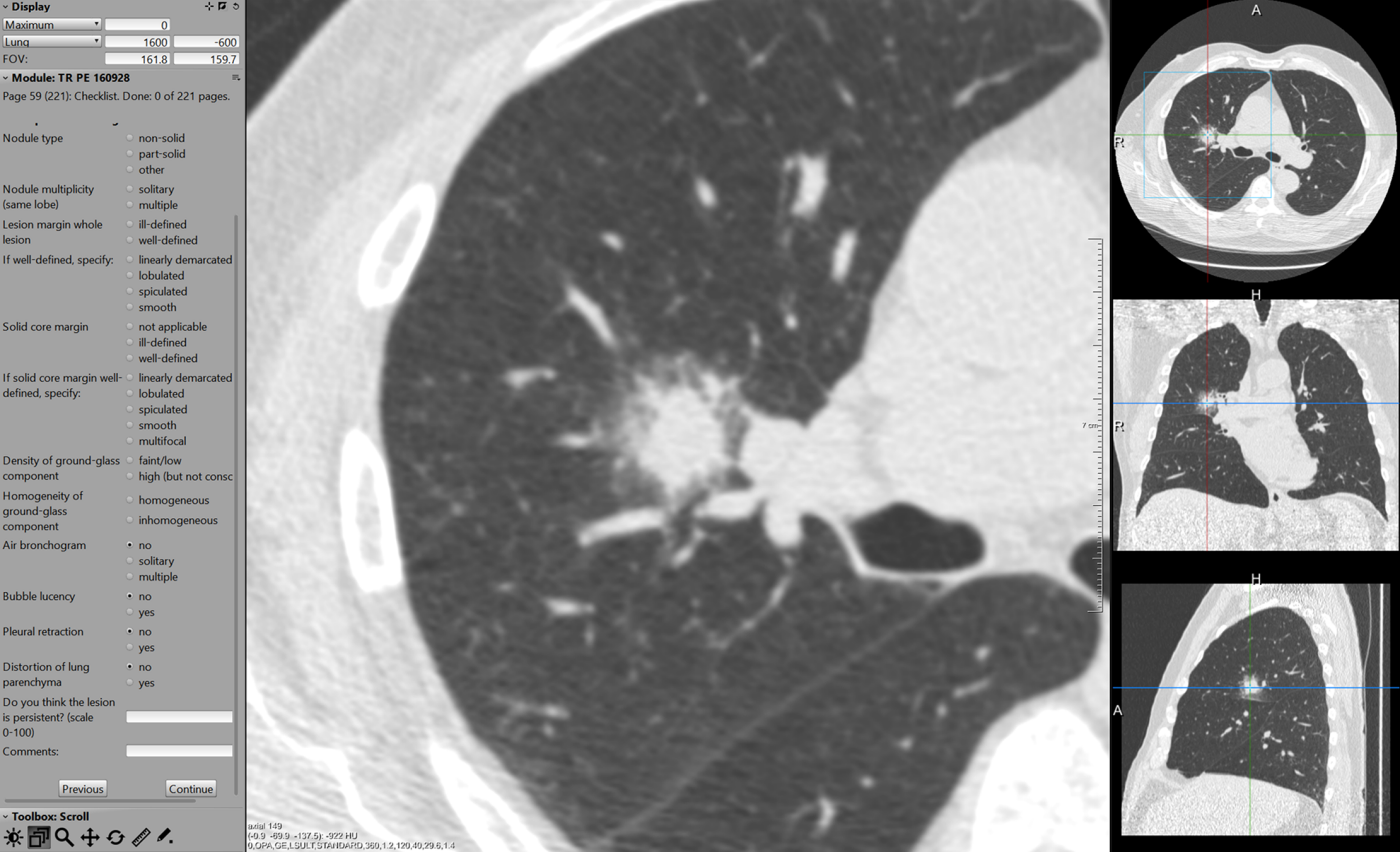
Reading workstation. The morphological features to be scored are listed on the left side of the monitor display. Lower-left corner has two text fields to enter the probability (0–100) and any comments. A magnified axial view of the nodule under evaluation is centered in the middle of the display. Coronal/sagittal projections are available on the right side of the screen, display size of the three projections was interchangeable. Processing tools such as windowing and magnification as well the full 3D CT dataset were available at any time.
